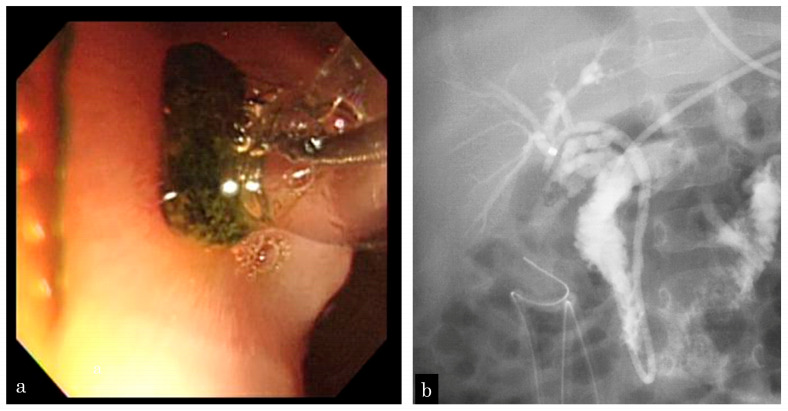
A 5-month-old girl who presented with vomiting and revealed gallbladder and common bile duct stones on ultrasonography. (a) EPBD is performed following recovery from cholecystitis and sepsis, and the stone is removed by one balloon pulling. (b) Following EPBD, a 5-Fr prophylactic ENBD tube is inserted, and no residual stone is revealed by cholangiography.
